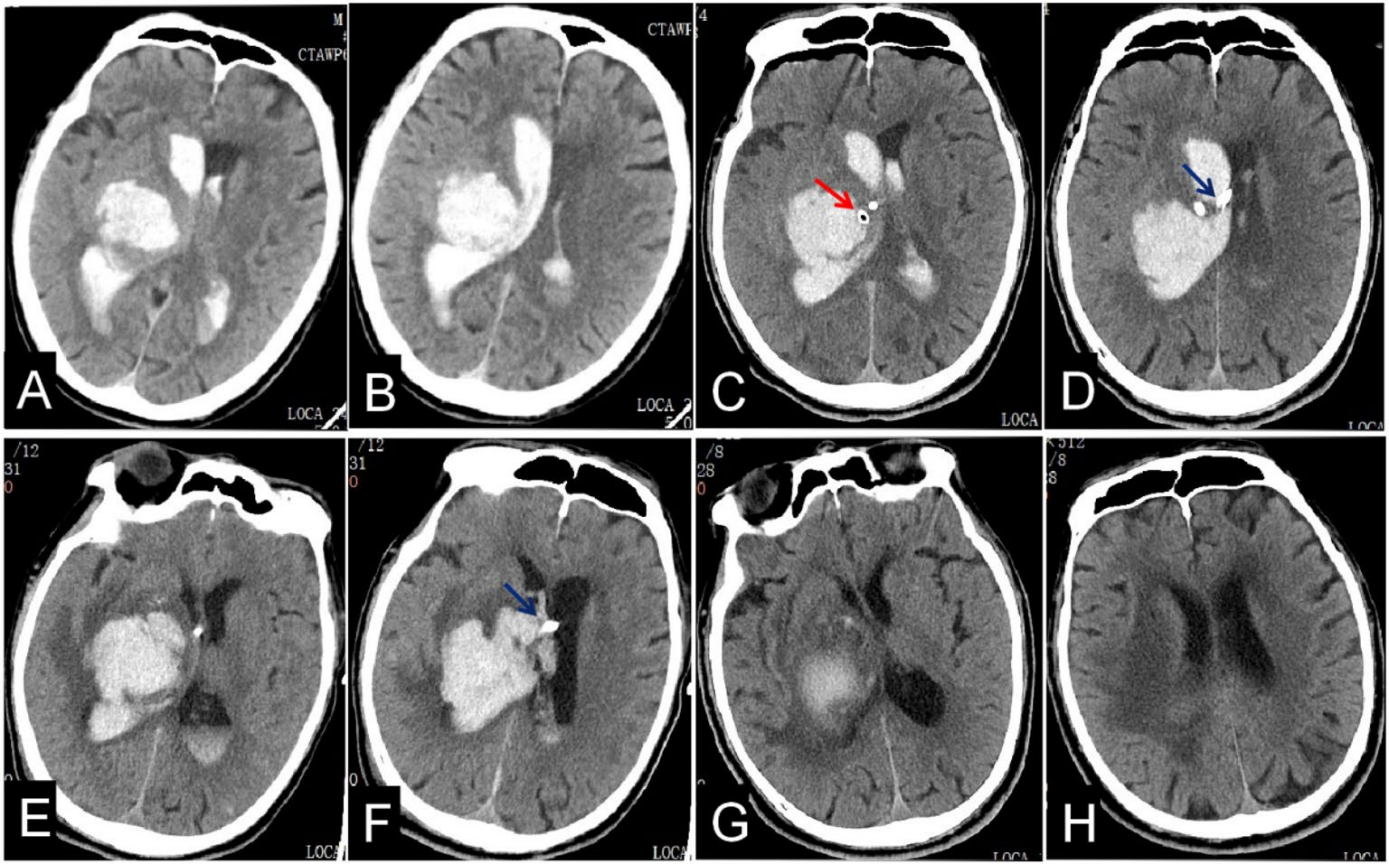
The misplacement of EVD catheter was shown by the CT scan. IVH was shown at 5 h pre-operation (A,B); The locations of the EVD catheters were shown at 1 d post-operation by freehand (the left catheter shown by the blue arrow in the contralateral ventricle, and the right catheter shown by the red one in the cerebral parenchyma, (C,D); The right catheter was removed next day after the CT scan post-operation (E,F); There was no hydrocephalus shown at 1 m follow-up, at the same time, uncomplete clearance of ICH (G,H).
